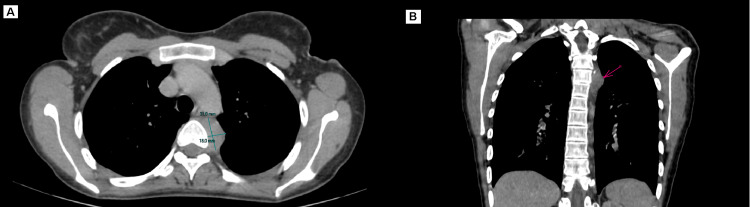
Axial (A) and Coronal (B) CT scan images (arrow) Showing a posterior mediastinal, retroesophageal and prevertebral mass at the level of D4, in close contact with the descending thoracic aorta and the vertebral body of D4.
